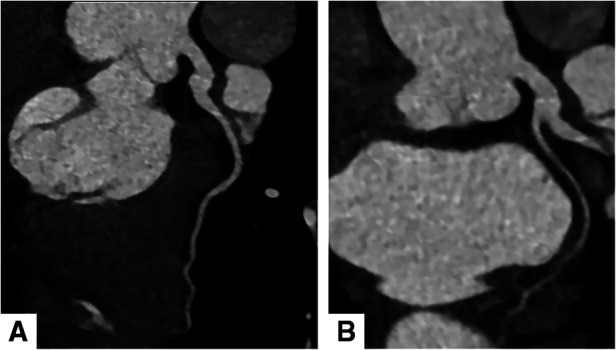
Computed tomography coronary angiography showed normal left anterior descending artery (A) and left circumflex artery (B).
